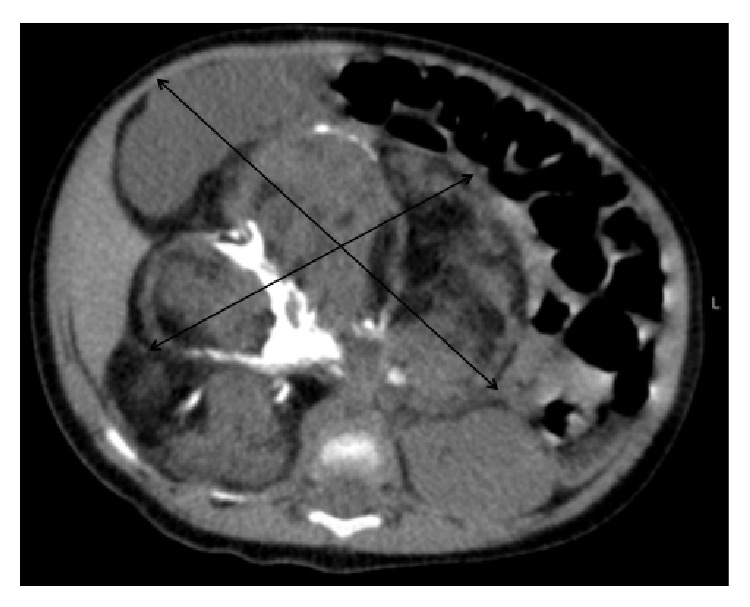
MRI of abdomen showing heterogeneous mass extending from the right suprarenal fossa and into the peritoneal cavity, measuring 10.2 cm × 10.5 cm × 8.5 cm (AP × TRV × SI). There are multiple large collections of high T2 signal consistent with cystic component degeneration.
